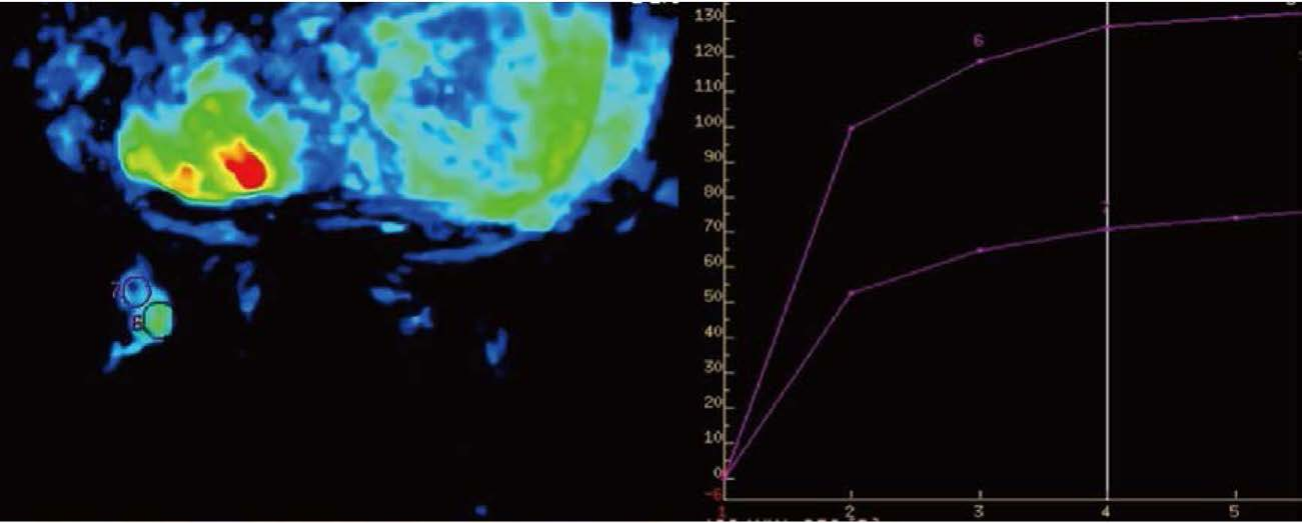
T1-weighted dynamic contrast-enhanced magnetic resonance imaging (DCE-MRI) of the left breast at the 3 o’clock position (2 fused masses) demonstrated a type II time curve.
